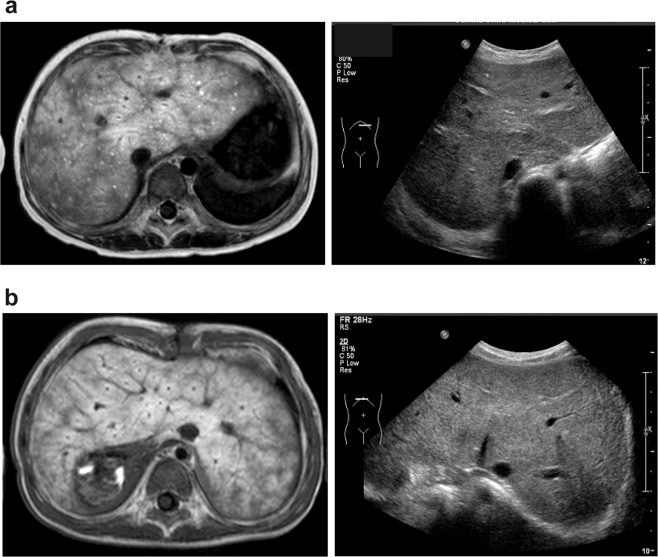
Comparison of gadolinium ethoxybenzyl diethylenetriaminepentaacetic acid (Gd-EOB-DTPA)-enhanced magnetic resonance imaging (i.e. EOB-MRI) and abdominal ultrasonography. (a) The patient is a 5-year-old girl with hypoplastic left heart syndrome. The left panel shows the EOB-MRI T1-weighted (T1WI) findings at 1 year after the Fontan procedure. A contrasting defect of the liver parenchyma, inhomogeneous enhancement in the liver periphery, and diffuse high-intensity spots are visible, which indicate cholestasis. In the right panel ultrasonography of the liver shows high echoic spots and a low echoic area. This finding reveals roughening of the liver parenchyma. (b) The patient is a 2-year-old boy with right isomerism heart and a single right ventricle. The left panel shows the EOB-MRI (T1WI) findings at 1 year after the Fontan procedure. Reticular contrasting defects of the liver parenchyma that correspond to the hepatic vein area are visible. Its appearance resembles “frog eggs.” The findings of “frog egg” is spread throughout the liver. The surface of liver is rough. The right panel shows the findings of ultrasonography of the liver, which has high echoic spots and a low echoic area.
